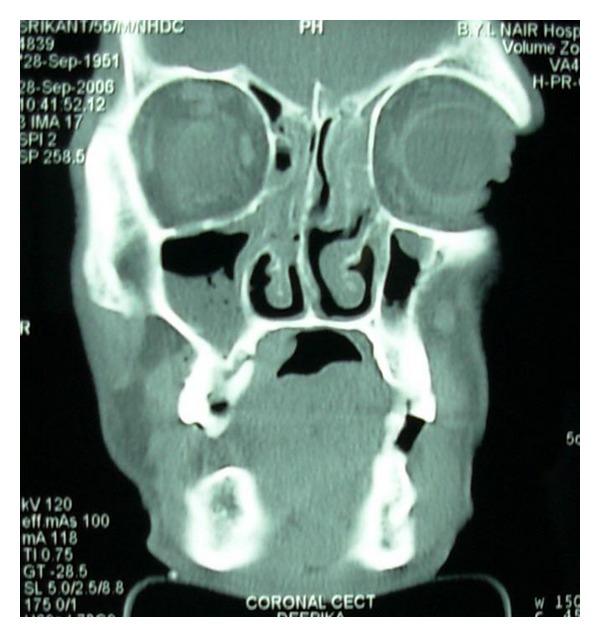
Coronal CT scan at the level of the maxillary right first molar showing bone destruction around the tooth.
